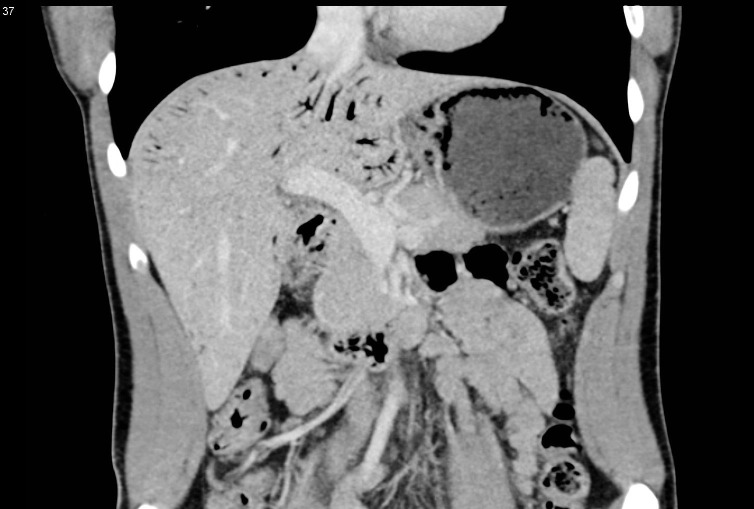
Coronal portal venous phase CT image showing gastric mucosal thickening and pneumatosis with portal venous gas.
